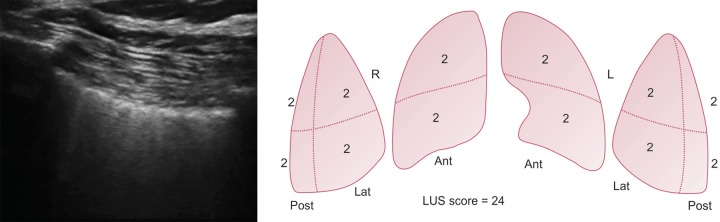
Ultrasound showing multiple B lines suggestive of interstitial syndrome with calculation of lung ultrasound score
